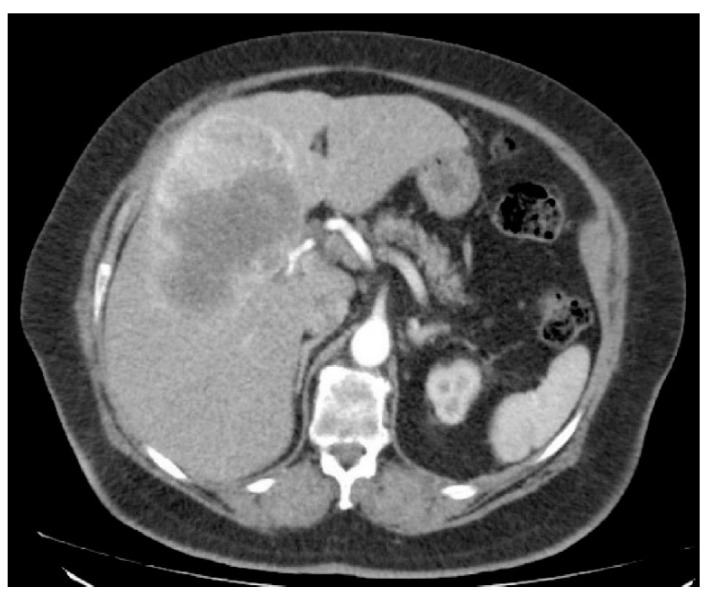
CT of a patient with PEComa. The arterial phase is associated with a strong, heterogeneous enhancement of contrast changes.
